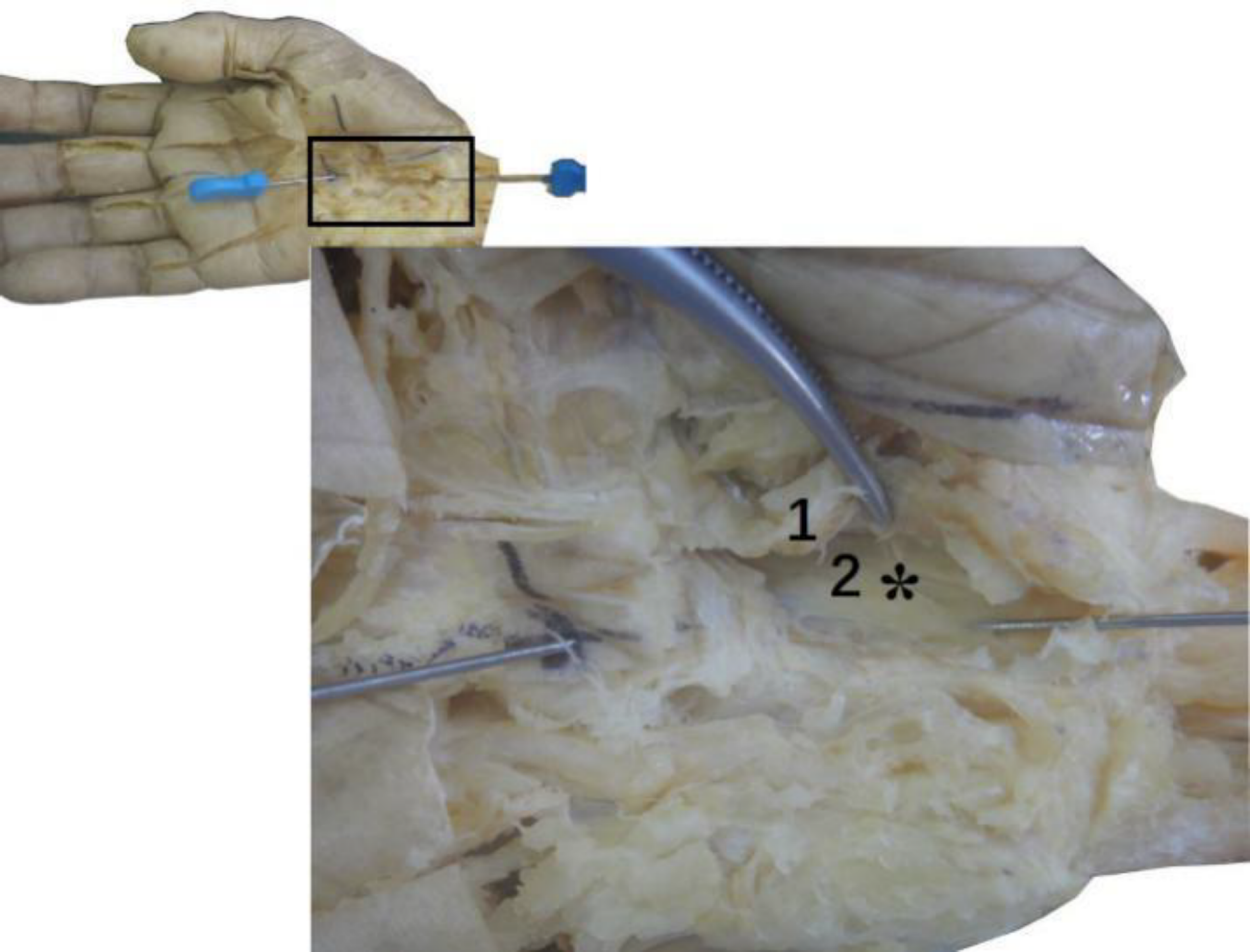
The median nerve was scratched by a needle knife in the non-ultrasound guided group. The asterisk refers to the cutting marks of the needle knife. 1, Transverse carpal ligament (turned up in section); 2, Median nerve.
